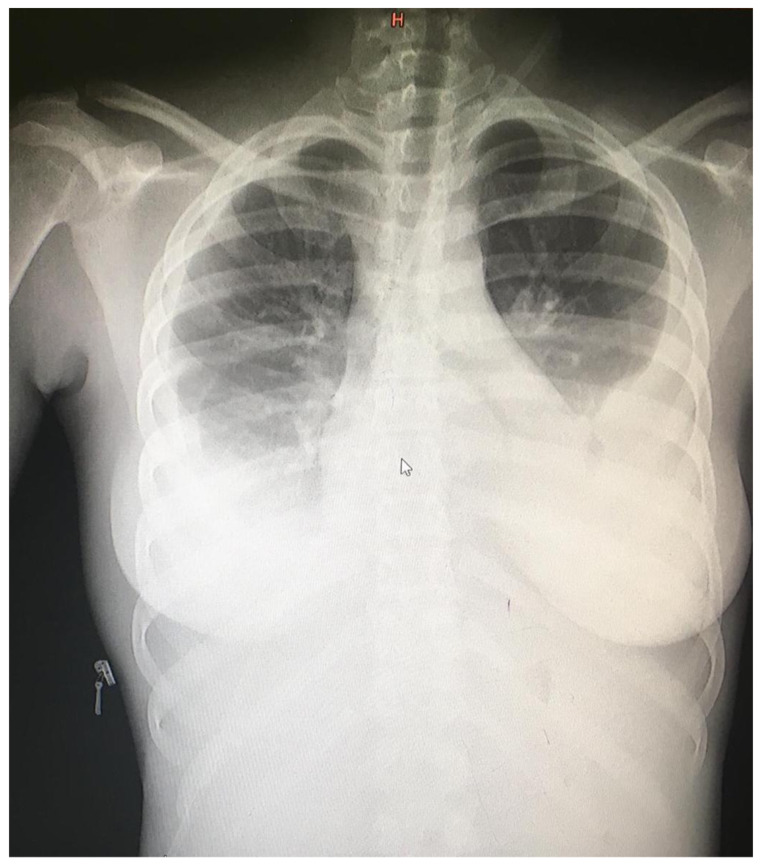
Chest radiograph (Day 0) showed bilateral pleural effusions and possible left lower-zone infiltrates.
